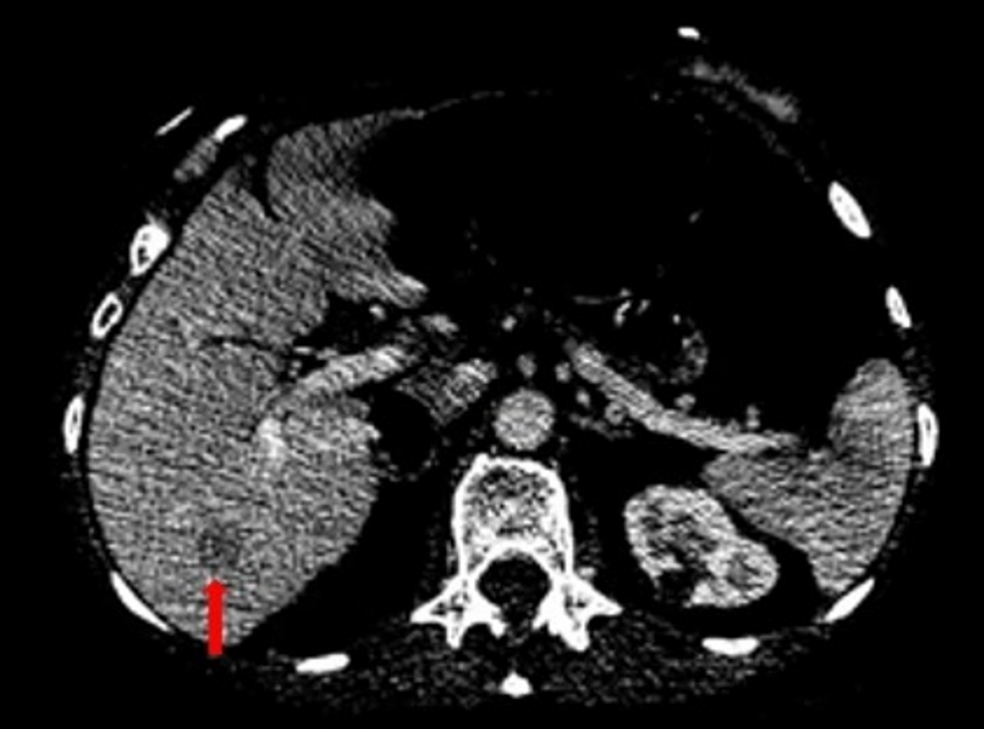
Selected axial section of the CT abdomen demonstrating a hypodense metastatic liver lesion (red arrow).
